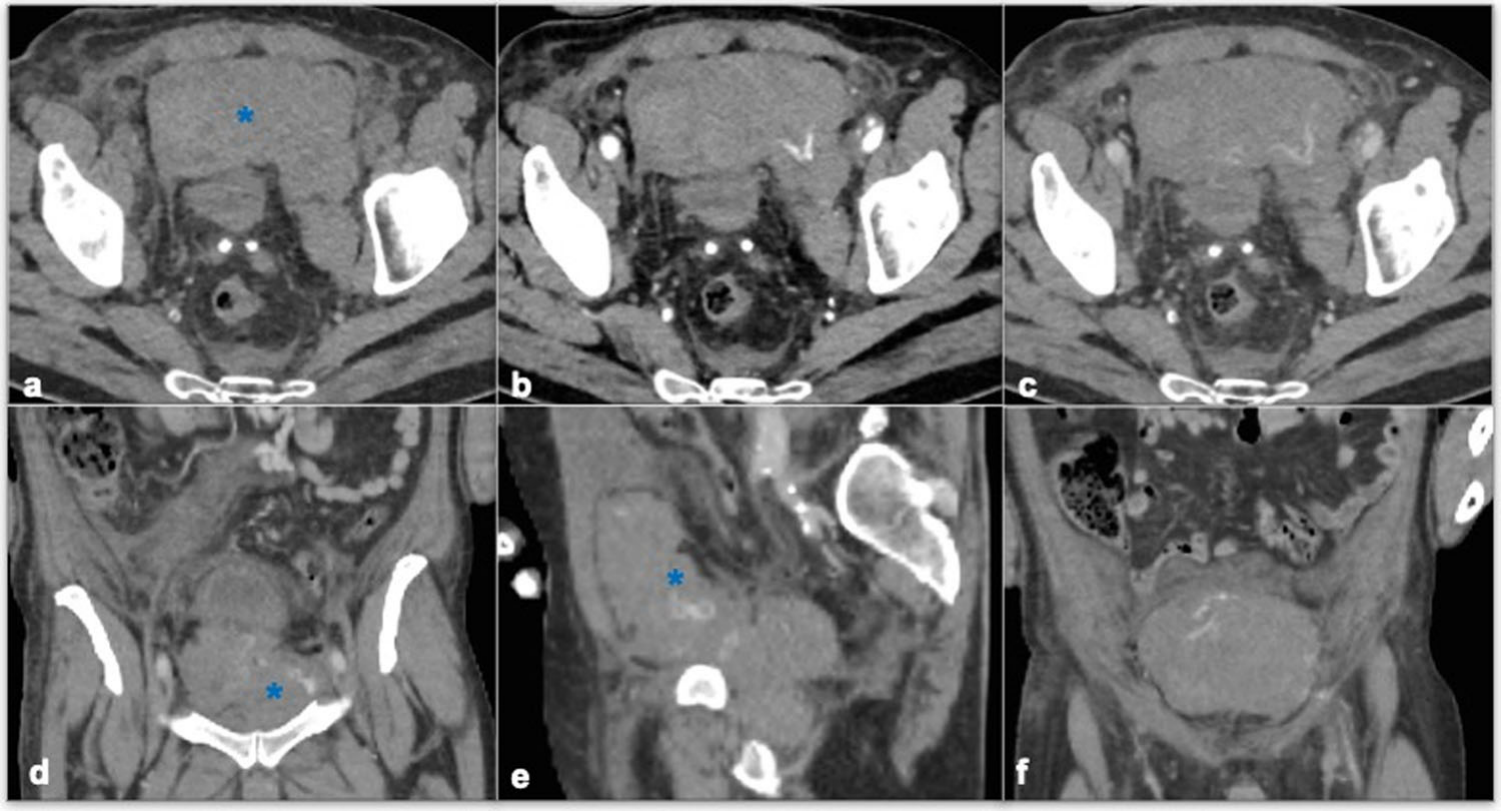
A 70-year-old patient subjected to TAVI 2 days before developed hypotension, anemia (Hb: 9 g/dL) and altered mental status. Contrast-enhanced CTA was performed to rule out acute bleeding, showing a massive lower abdominal spontaneously hyperdense collection (a) (asterisk, mean HU = 52), with active bleeding inside (b, arrow, arterial phase) (c, arrow, venous phase), as a result of an iatrogenic rupture of an arterial femoral-iliac vessel on the left (d, e asterisks) of 6 × 20 × 8.5 cm (a, f)
